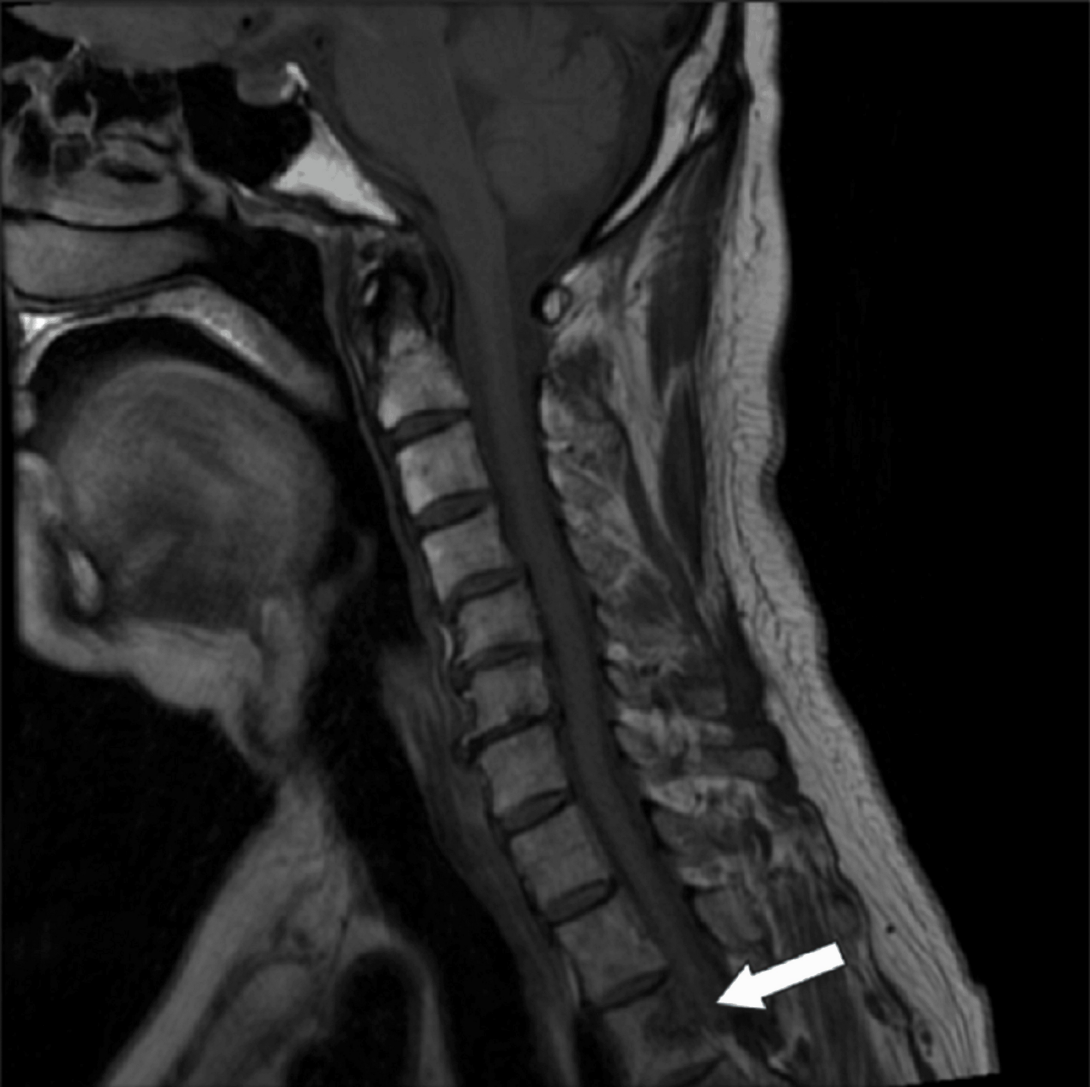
Metastatic sites in patient 1 Magnetic resonance imaging demonstrated thoracic vertebral metastasis, as indicated by arrows.
